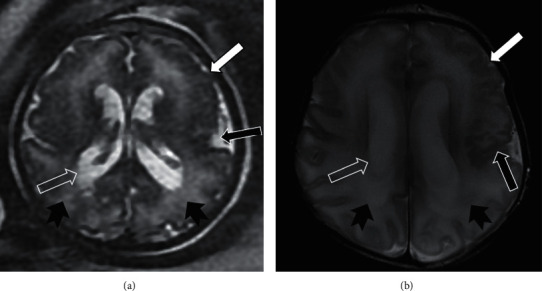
CMV infection. Gestational age: 35 weeks. (a) Fetal Axial SSFSE T2. (b) Postnatal cerebral MRI at 8 days of life, axial FSE T2. The presence of diffuse affectation of the white matter of parietal predominance (solid black arrowhead) with mild ventriculomegaly (empty white arrow) is shown. Fetal MRI provided useful information for perinatal management and prognosis over fetal ultrasound by detecting left frontal (solid white arrow) and left Sylvian fissure (solid black arrow) polymicrogyria not visible in the fetal ultrasound.
